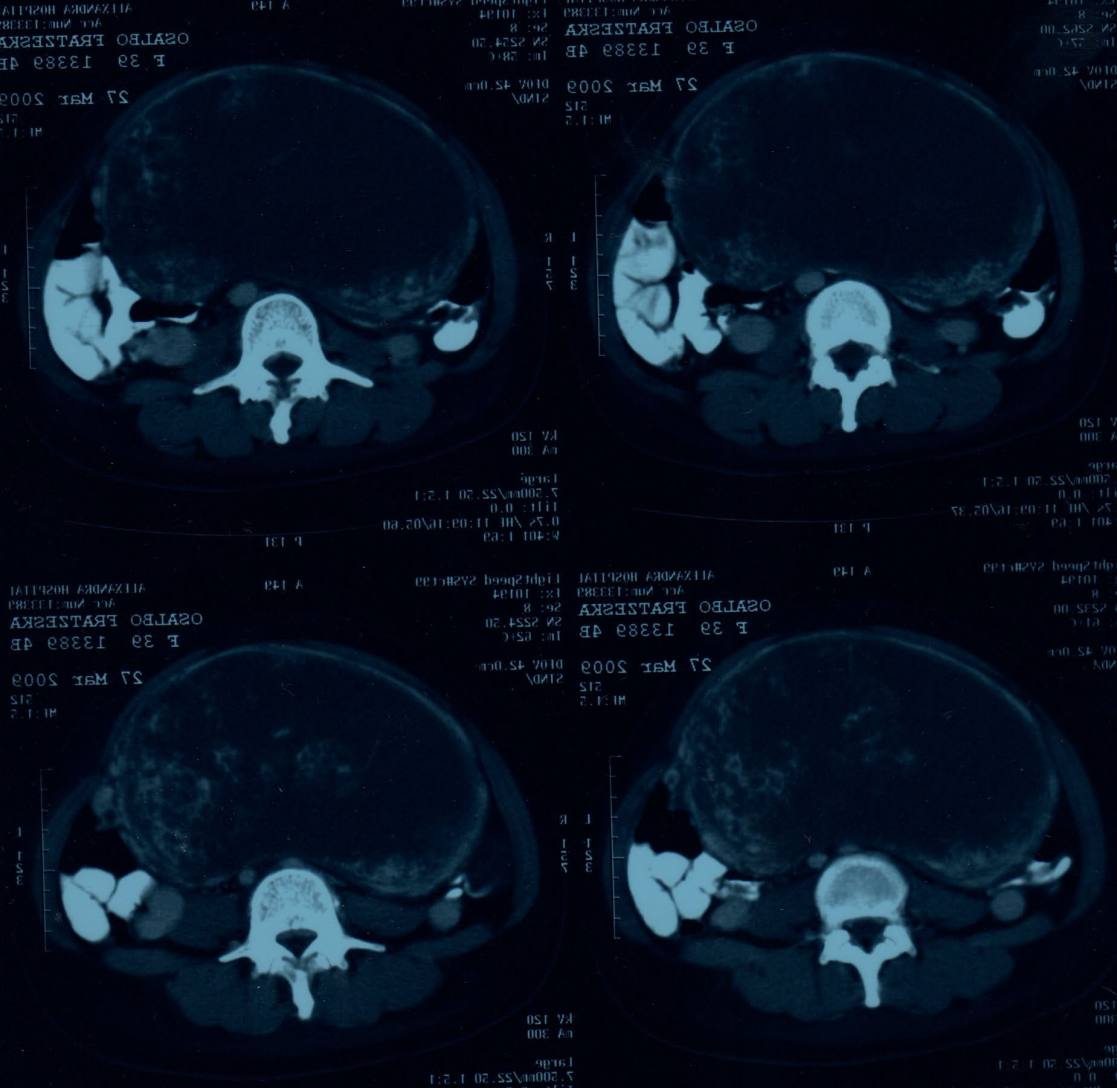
Abdominal CT showing occupation of the uterus by the trophoblastic tissue.
